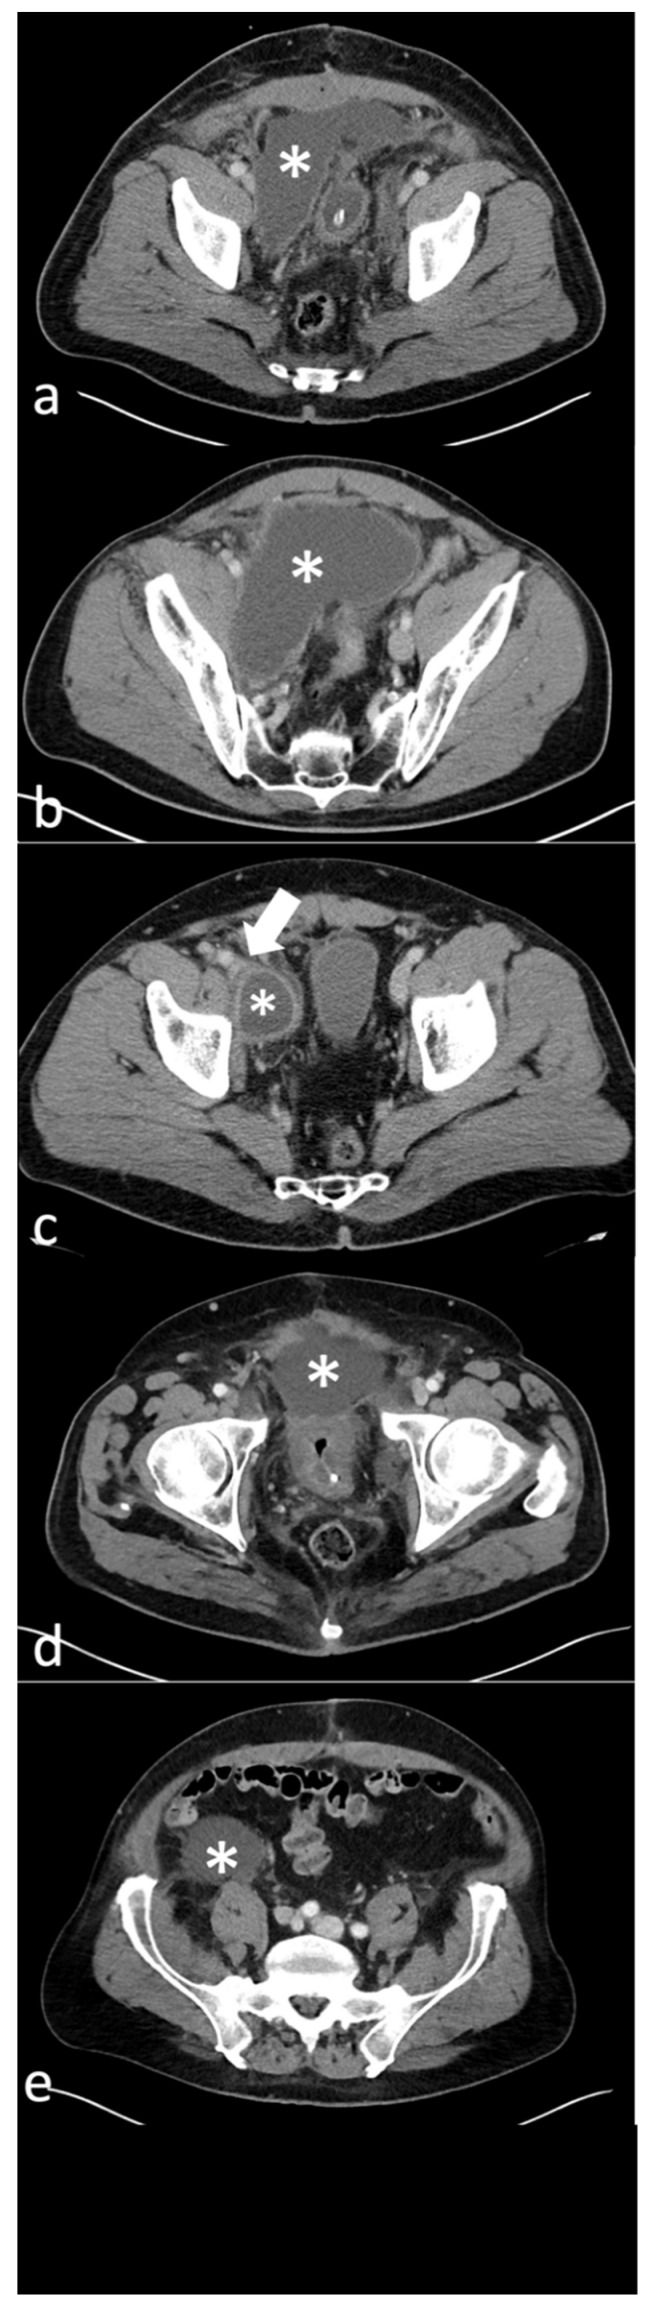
(a)-Axial CT image of a patient with a Type 1 lymphocele (*), 9 days after radical prostatectomy with pelvic lymph node dissection. (b)-Axial CT image of another patient with a large Type 2A lymphocele (*), 51 days after radical prostatectomy with pelvic lymph node dissection. (c)-Axial CT image of a different patient with a right-sided Type 2B lymphocele (*), 4.5 months after radical prostatectomy with pelvic lymph node dissection. The lymphocele is seen to compress the right external iliac vein (arrow) and shows peripheral enhancement with mild surrounding fat-stranding. (d)-Axial CT image of another patient with Type 3(*) + 2A lymphocele, 22 days after radical prostatectomy with pelvic lymph node dissection. (e)-Axial CT image of a further patient showing a right retroperitoneal component of a Type 4 lymphocele (*), 16 days after radical prostatectomy with pelvic lymph node dissection.
